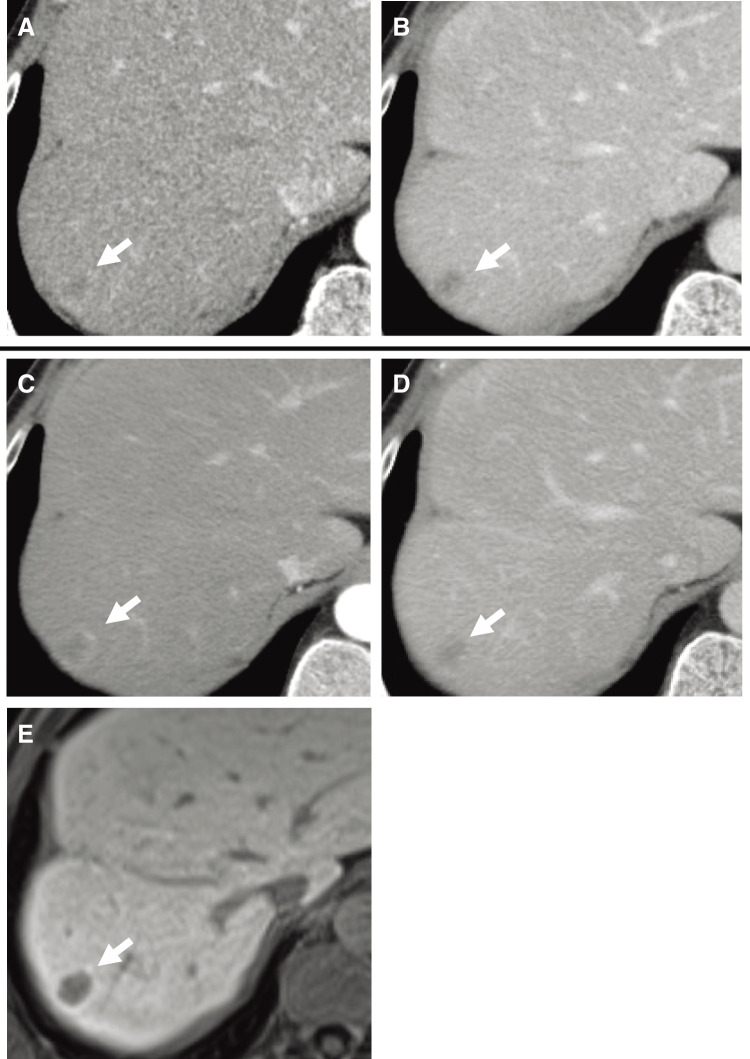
Imaging findings of recurrent HEHE (white arrow). Recurrent HEHE at diagnosis in (A) arterial phase and (B) portal phase of CT. (C) Recurrent HEHE after 5-months’ observation in arterial phase, (D) portal phase of CT, and (E) hepatobiliary phase of EOB-MRI. CT, computed tomography; EOB-MRI, ethoxybenzyl-diethylene-triaminepentaacetic acid-enhanced MRI; HEHE, hepatic epithelioid hemangioendothelioma; MRI, magnetic resonance imaging
